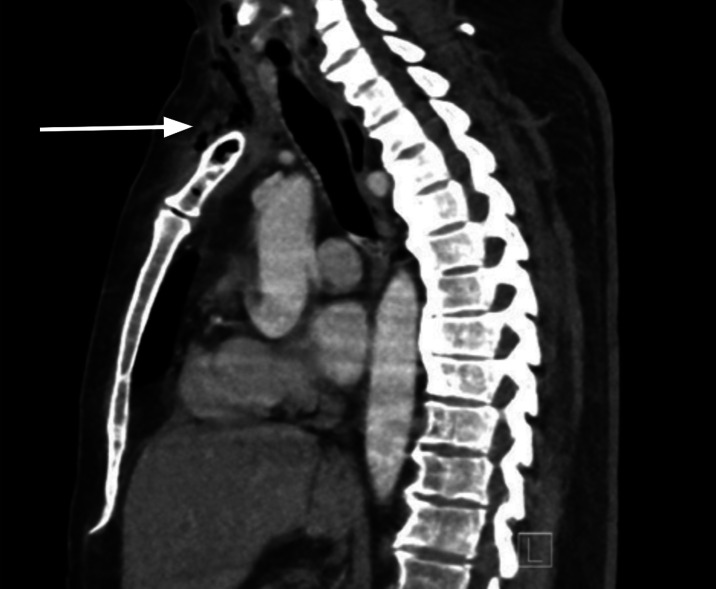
A sagittal view of computed tomography of the chest, revealing osseous infection of manubrium with surrounding gas (arrow) extending into the neck.
